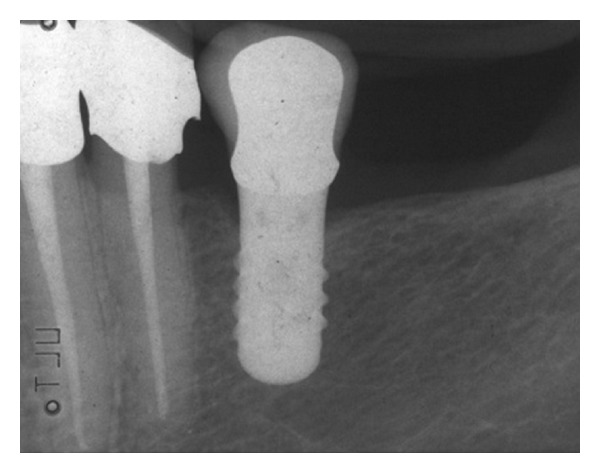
Single-tooth fixed prosthesis supported by a short implant (4.1 × 8 mm), 0 years loading. Periapical radiograph.
